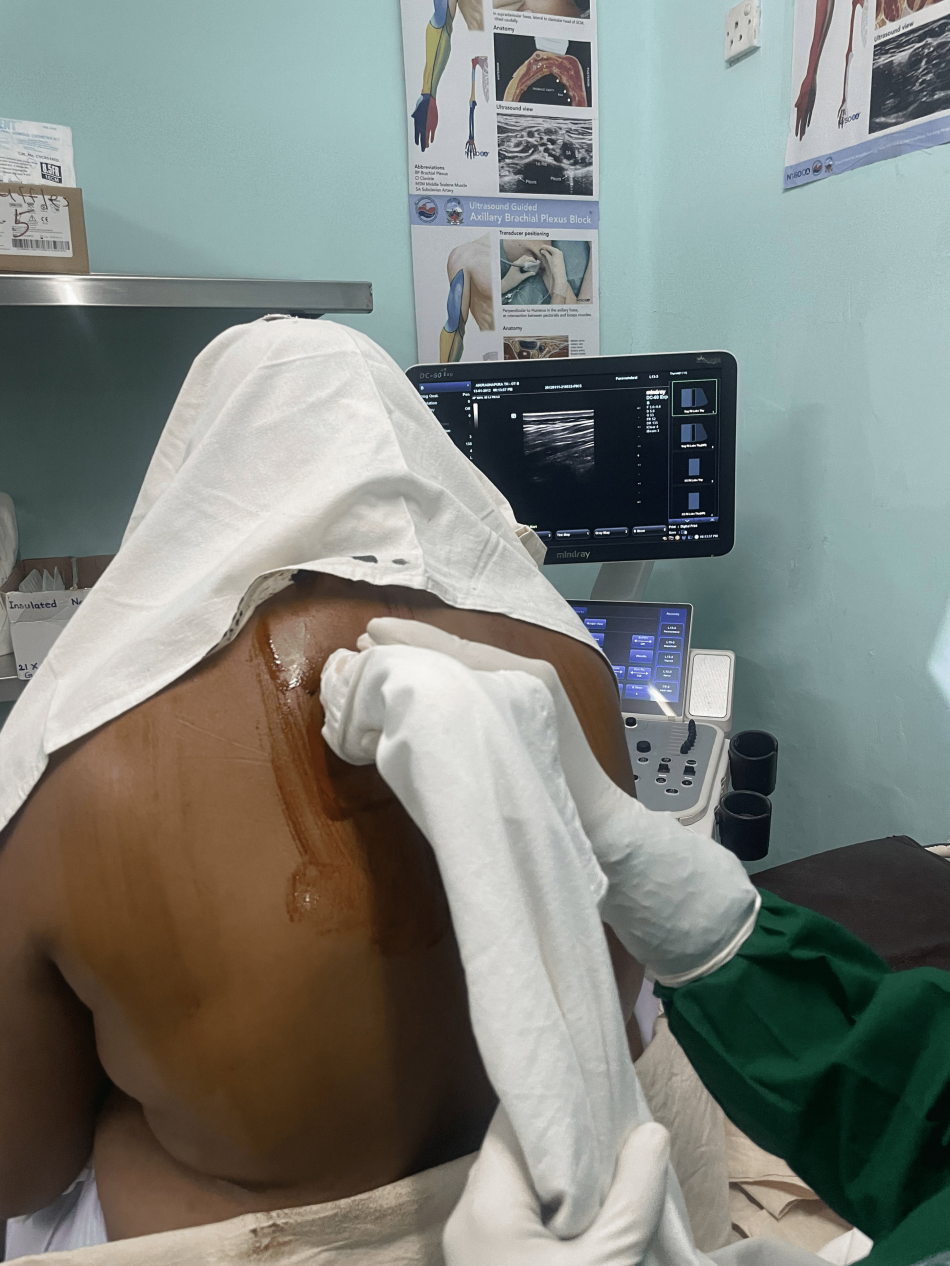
Ultrasound-guided paravertebral block being administered
